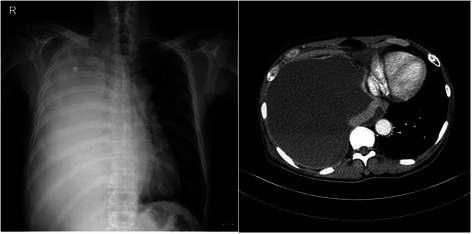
Initial chest x-ray and CT show that massive pleural effusion in the right hemithorax combined with diffuse pleural thickening and several small nodules in the left lung, suggesting malignant pleural effusion
